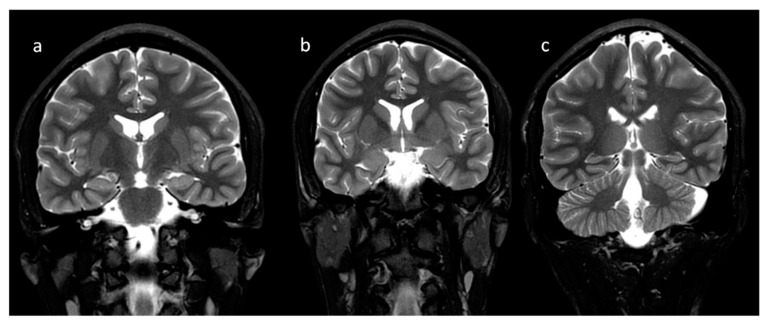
Completion of myelination on brain MRI and differences in signal intensity between the basal ganglia and the surrounding brain—coronal T2-WI; putamen and caudate nucleus head (a,b); thalamus and caudate body (c).
